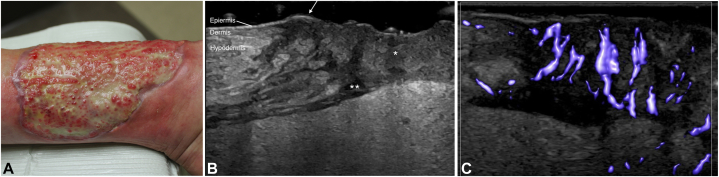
A, Healing PG ulceration on left lower extremity. B, HFUS of edge of ulcer. There is a hypoechoic wound bed (∗) with an edematous drainage tract deep to the ulcer (∗∗). At the wound edge, there is normal hyperechoic epidermis, slightly hypoechoic dermis, and subcutaneous layer without evidence of interstitial edema. The thin hyperehcoic epidermis extends from normal tissue at the ulcer edge over the wound bed (*arrow*). C, HFUS microvascular imaging shows moderate vascularity with organized, linear pattern in wound bed. *HFUS*, High frequency ultrasound; *PG*, pyoderma gangrenosum.
